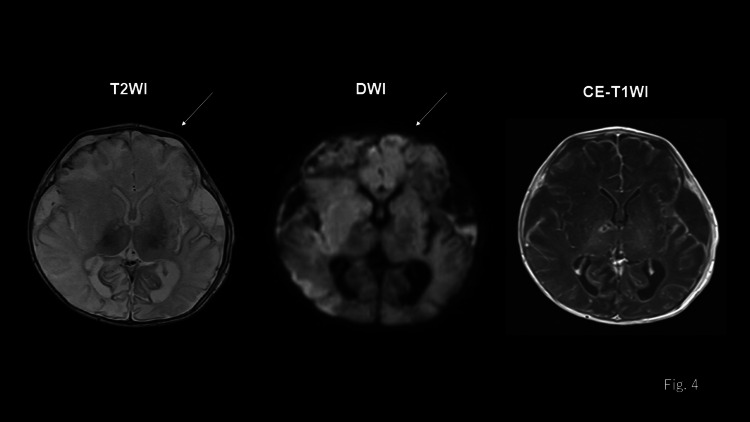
MRI of the brain on day 11 of hospitalization Bilateral subdural fluid collections in the frontotemporal regions revealed on a T2-weighted image.
